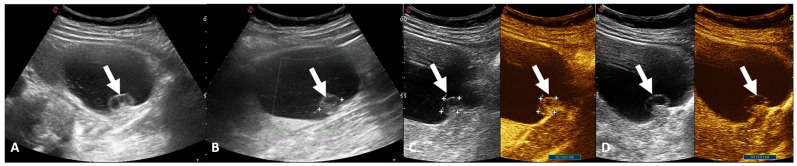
Renal ultrasound examination, B-mode (A,B) and CEUS (C,D) scans. Renal cystic lesion with small iso-hypoechoic wall nodule (arrows). After an intravenous contrast injection, the nodule shows enhancement. These features are suggestive of Bosniak Class IV.
